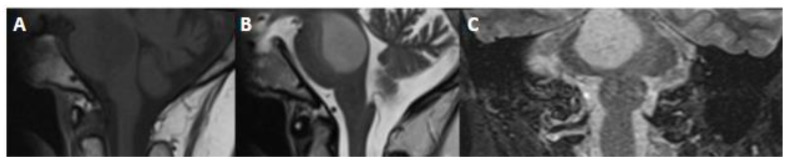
Sagittal T1-weighted (A), sagittal T2-weighted (B), and coronal T2-weighted short tau inversion recovery (STIR) cervical spine images (C) in a 32 year old woman with previously treated intracranial germinoma who presented with left sensorineural deficits. Images demonstrate an expansile, T1 hypointense and T2 hyperintense central pontine mass. This was diffuse pediatric-type high-grade glioma, H3-wildtype, receptor tyrosine kinase (RTK1) subtype, subclass C. RTK1 subtypes typically involve the supratentorial brain, with approximately 18% located in infratentorial/brainstem structures. Gliomas arising after therapeutic radiation, as in this patient, are predominantly of the RTK1 molecular subtype.
